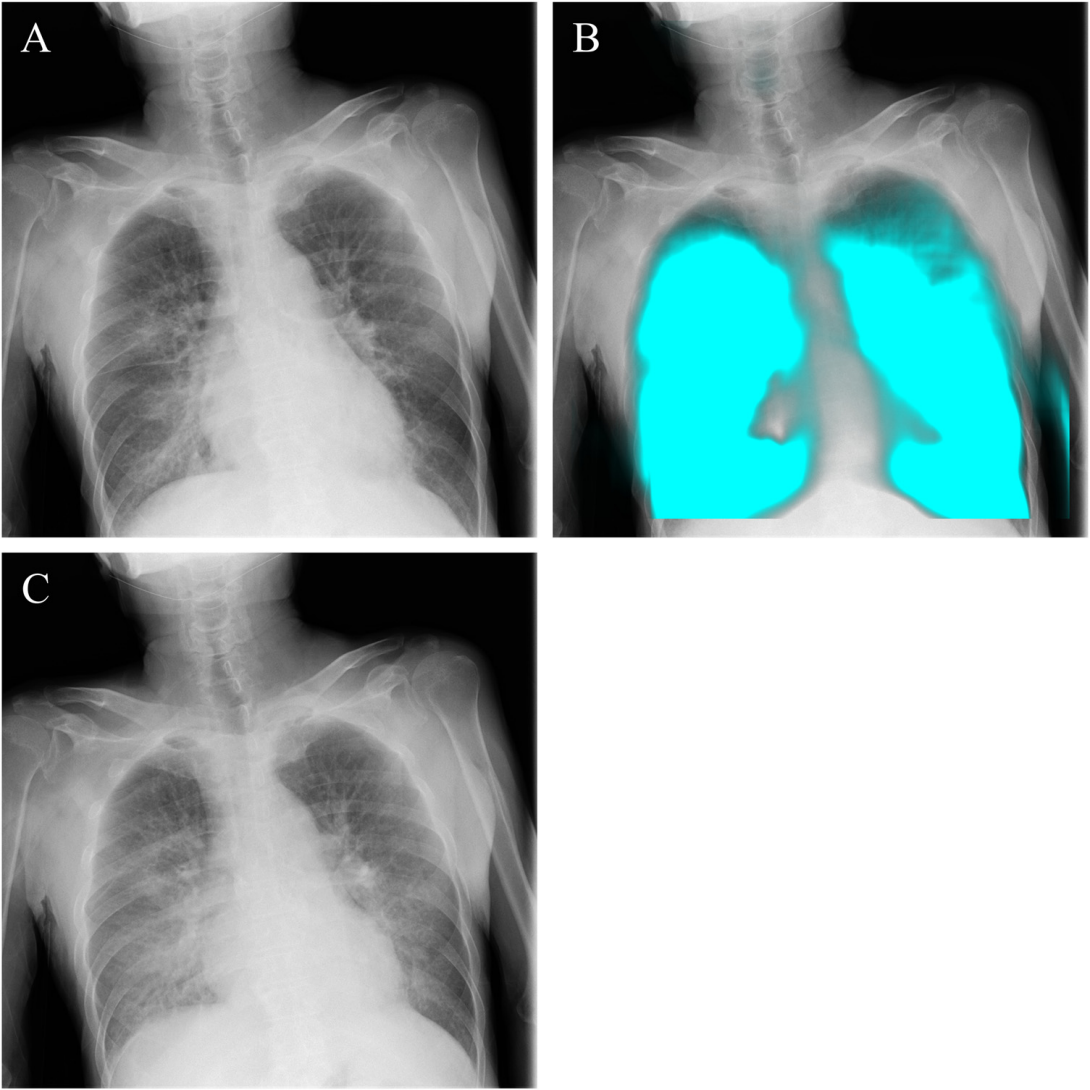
Dynamic chest radiography in a patient with pulmonary congestion. In the original images of dynamic chest radiography, increased lung translucency was observed at maximum inspiration (A), while pulmonary artery dilation and pulmonary congestion were noted at maximum expiration (C). In the PL-MODE image at maximum inspiration (B), a distinct signal increase associated with respiration was observed, suggesting good ventilation.
